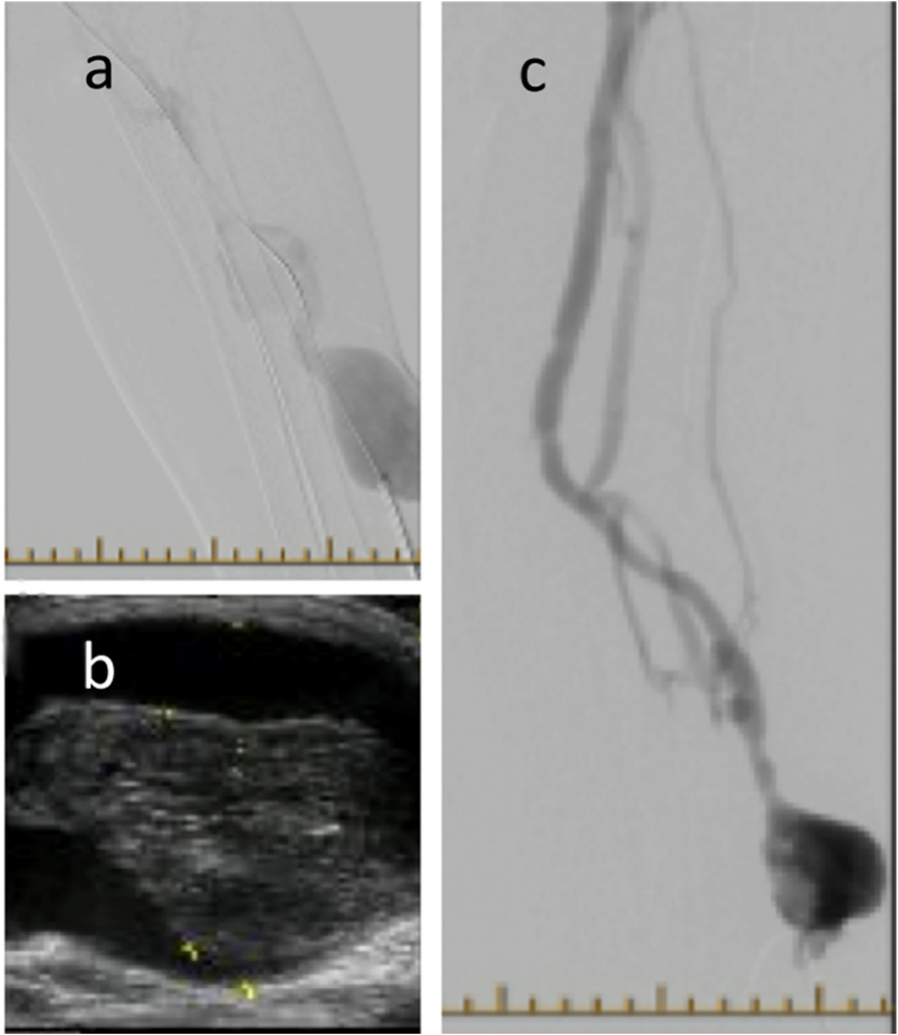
Fistulogram and ultrasound images of radio-cephalic fistula. a: Fistulogram after attempted angioplasty and before tPA infusion. Clot is seen in the venous aneurysm. b: Ultrasound demonstrates a persistent large clot in the venous aneurysm. c: Fistulogram of venous aneurysm one week after tPA infusion, demonstrating significant decrease in clot burden.
